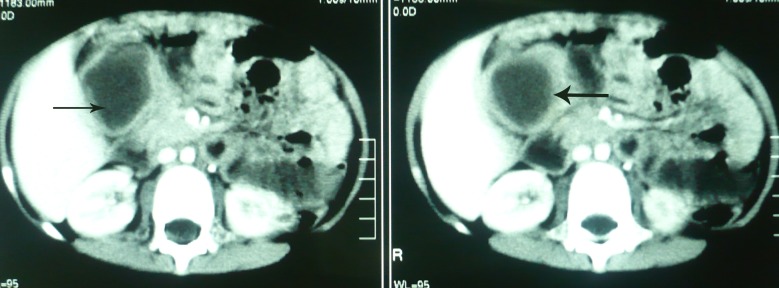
Figure 1: CT scan of the abdomen showing a hypo-dense cyst with wall enhancement.
